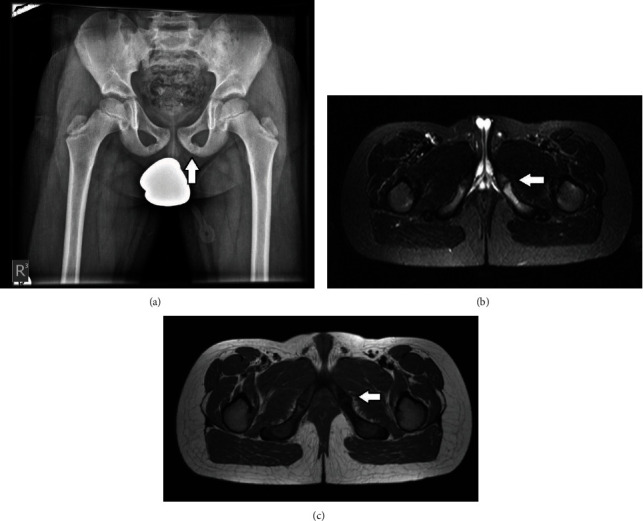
(a) Asymmetric enlargement of the left ischiopubic synchondrosis. (b and c) STIR (b) and T1W (c) sequences in transverse plane in an adolescent with left gluteal pain, demonstrate irregularity of the left ischiopubic synchondrosis, with bone marrow edema involving the bony structures around the synchondrosis. The adjacent soft tissues maintain their normal MRI signal.
